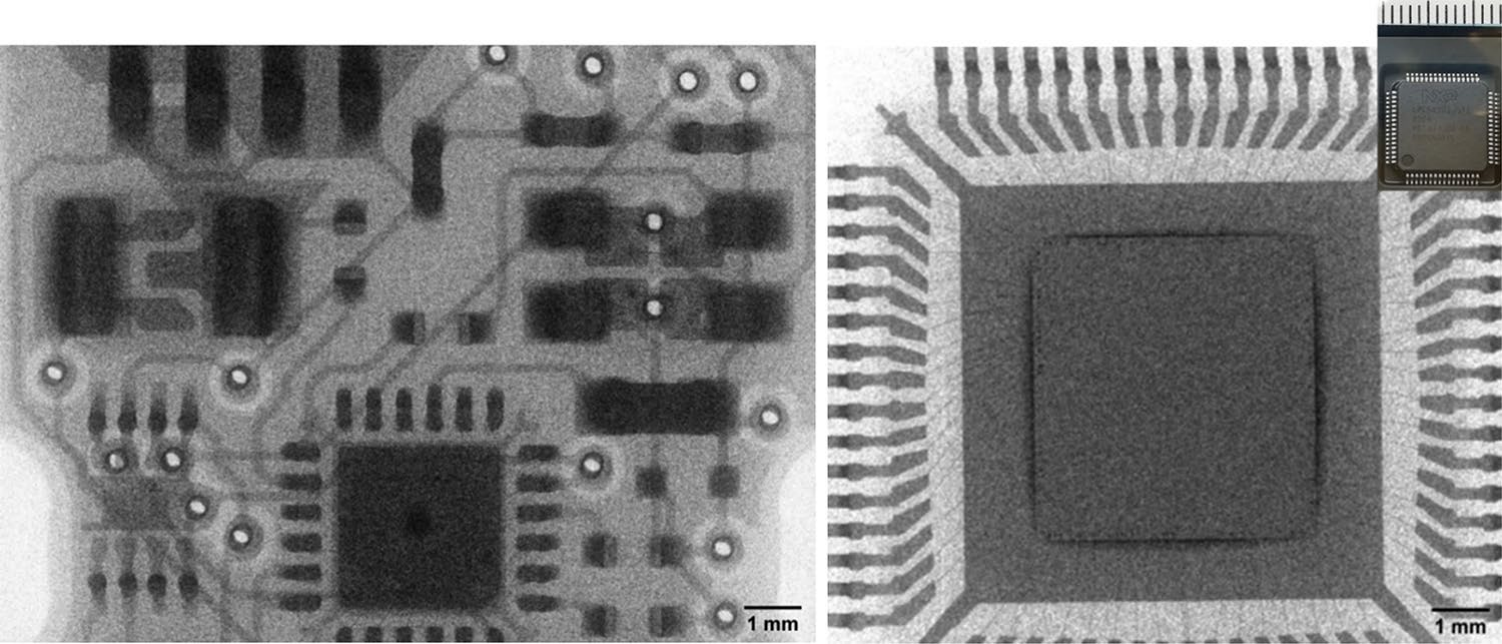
X-ray images of an USB-drive circuit and a microchip. The inset shows the physical mm-scale microchip.
